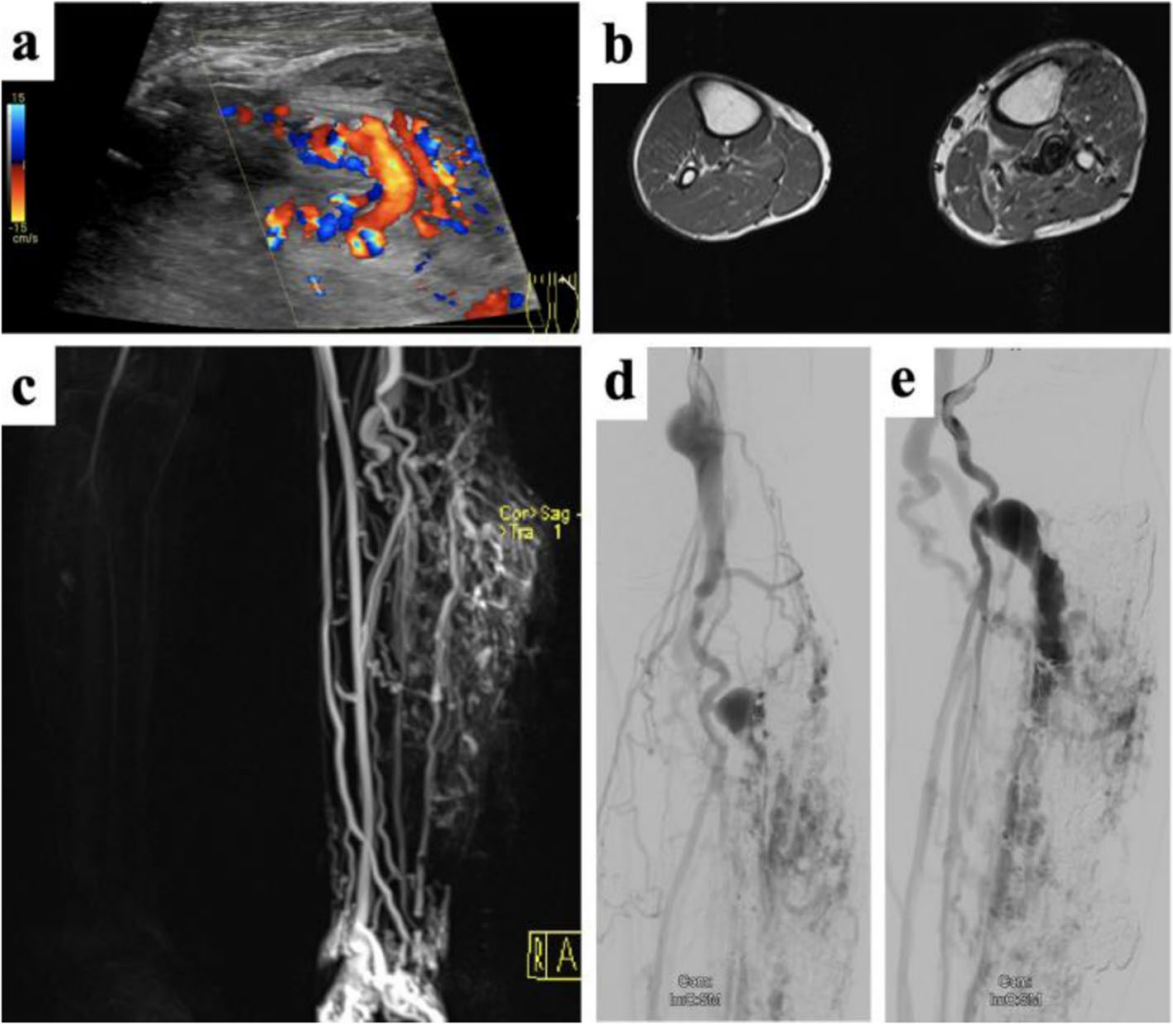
Arteriovenous malformation of the lower limb with associated soft-tissue proliferation. a Color-coded Duplex ultrasound reveals vigorous flow along the AVM nidus. b Cross-sectional MRI reveals multiple flow-voids as sign of arterialized flow patterns together with soft-tissue proliferation adjacent to the AVM. The mass was clinically pulsatile without ulceration at the time of clinical presentation. c Dynamic MR angiography shows rapid filling of the AVM along the left lower limb at time with only initial enhancement of the arteries of the non-affected leg. Pre-embolization angiogram in the late arterial phase (d) and early venous phase (e) depicting dilated aneurysms along the venous outflow tract
